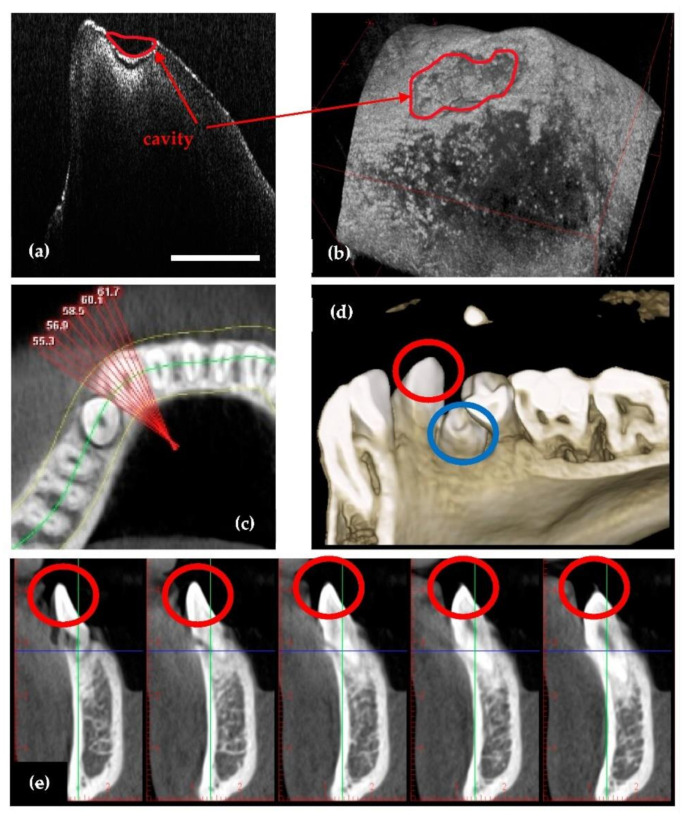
Example of a case where OCT spots a small cavity on the enamel level of the tooth: (a) OCT B-scan; (b) OCT 3D reconstruction; (c) 3D CBCT axial view of the tooth; (d) 3D rendering of CBCT; (e) 3D CBCT sagittal view of the tooth (the latter taken at different, successive depths into the hard tissue). Scale: 1 mm.
